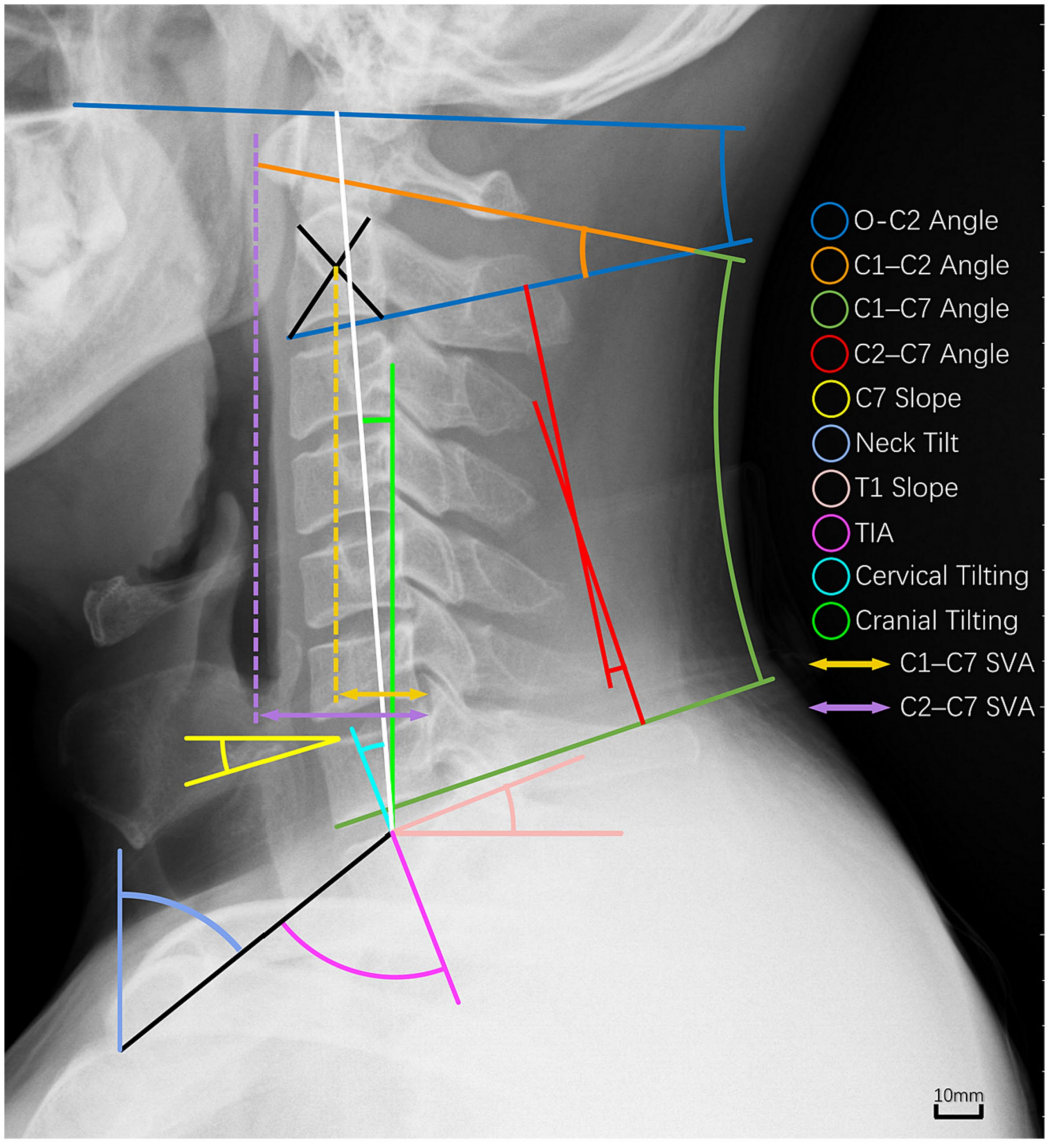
Measured cervical sagittal parameters of the study in the lateral cervical radiograph.
